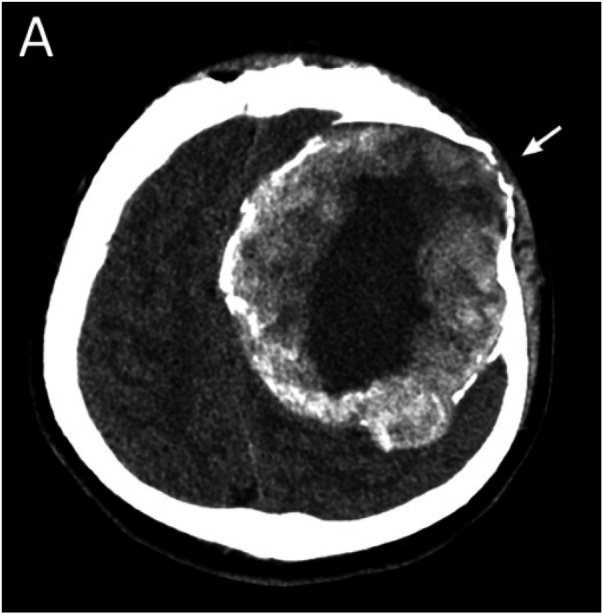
Early postoperative CT after pseudotumor excision. Axial CT shows the left frontoparietal postoperative cavity and skull defect (arrow).
